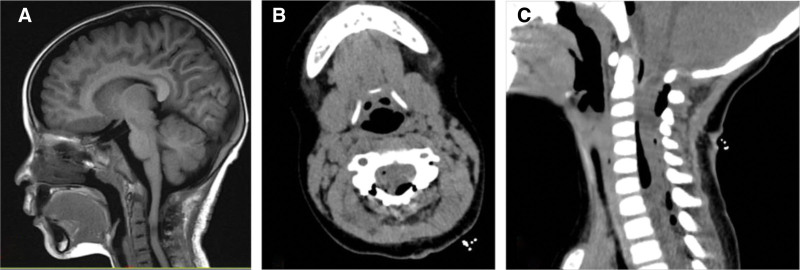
Postoperative imaging review results. (A) Postoperative sagittal T2-weighted magnetic resonance imaging showed complete resection of the lesion and significant relief of spinal cord compression. (B and C) Axial computed tomography and sagittal reconstruction images demonstrated good repositioning of the vertebral lamina, satisfactory reconstruction of the spinal canal structure, and no residual lesions were seen in the surgical area. T2 = Transverse Relaxation Time.
